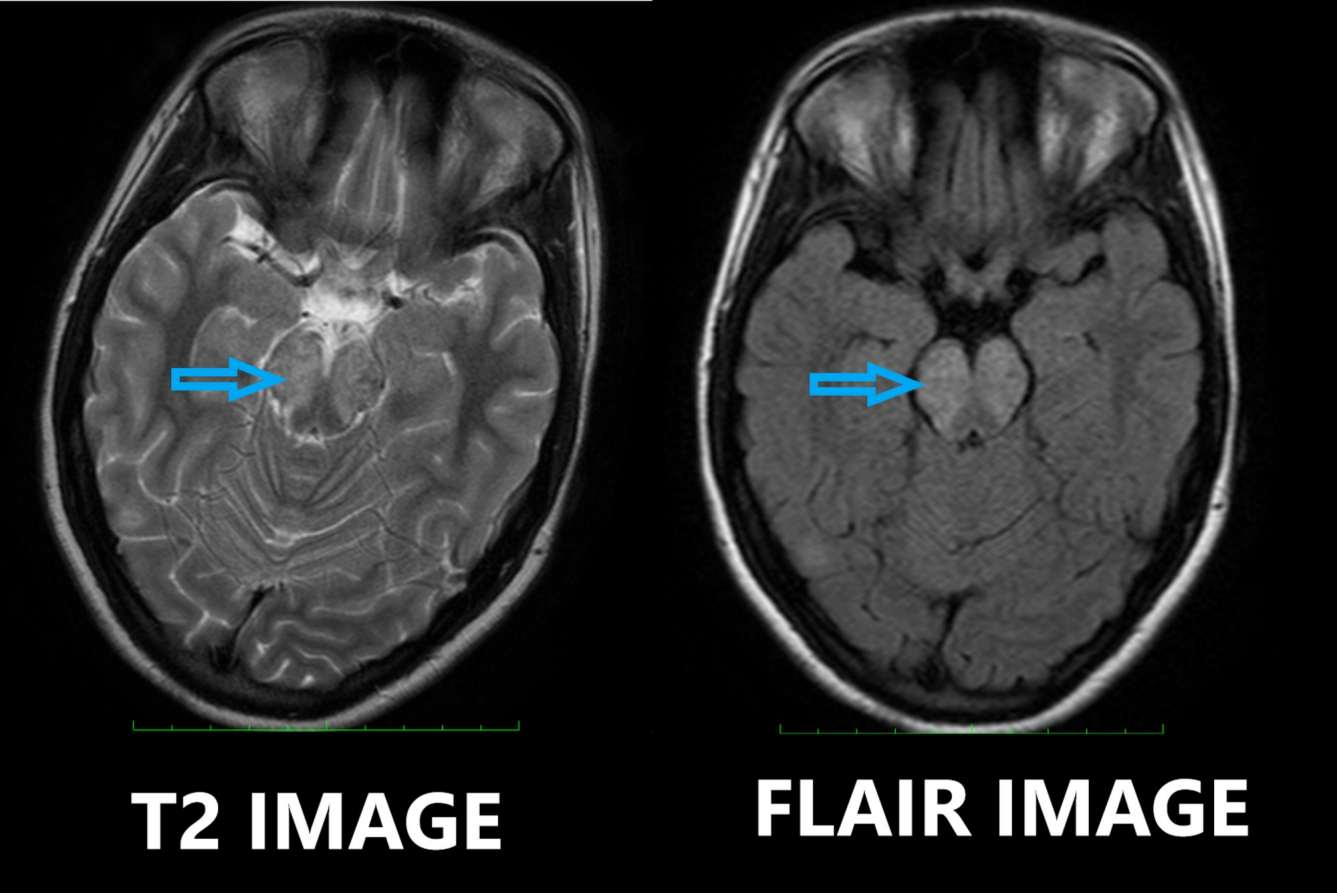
T2-weighted and fluid-attenuated inversion recovery (T2-FLAIR) sequence axial MRI images showing punctate lesions in the pons (blue arrow). MRI: magnetic resonance imaging
